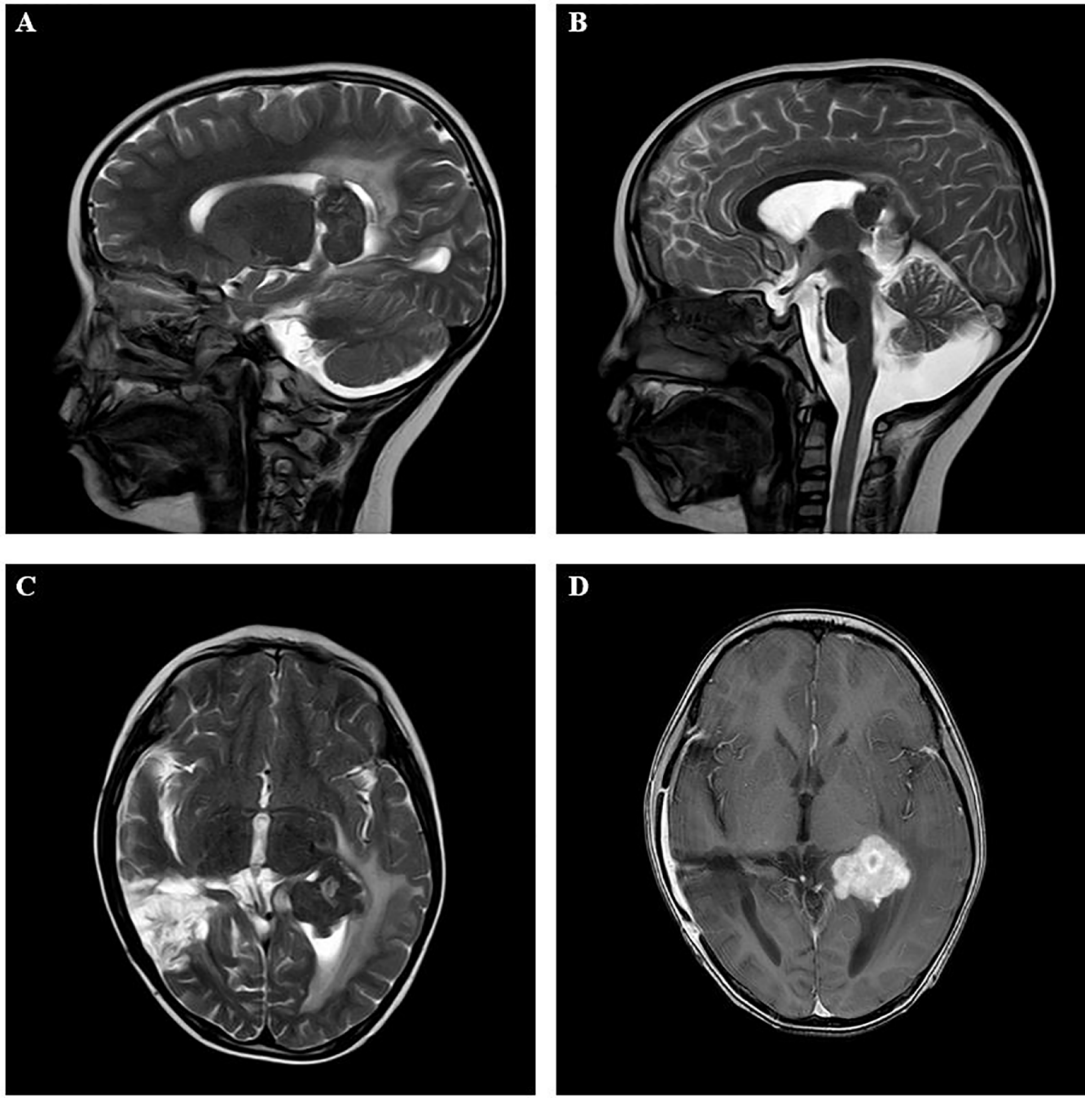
Imaging results in the intracranial lesion tissue. The results of MRI showed the lateral ventricle expansion, with abnormal signal mass shadow (size about 3.3×3.1×3.5cm) in the lateral ventricle. (A, B: sagittal position; C, D: horizontal position). MRI, Magnetic resonance imaging.
